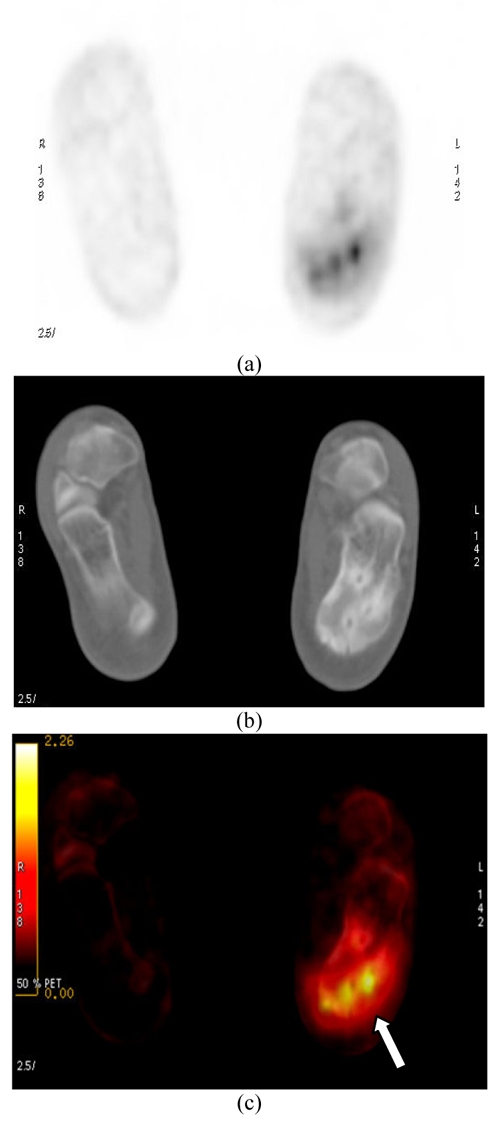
(a) Coronal PET image showing foci of avid ^18^F-FDG intensity signal lesions within the right calcaneum; (b) Coronal low-dose CT image showing foci of low attenuation lesions with rim of sclerosis; (c) A combined PET/CT image displays foci of avid ^18^F-FDG uptake at the sclerotic rims of the right calcaneum extending to the adjacent inferior cortex (arrow).
